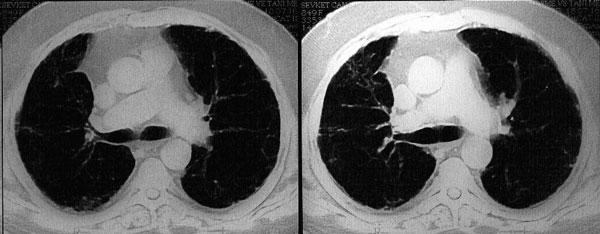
Repeat HRCT of patient performed after eleven months discontinuation of PEG-IFN and ribavirin with systemic steroid showing resolution (improvement) of the ground glass opacities.
